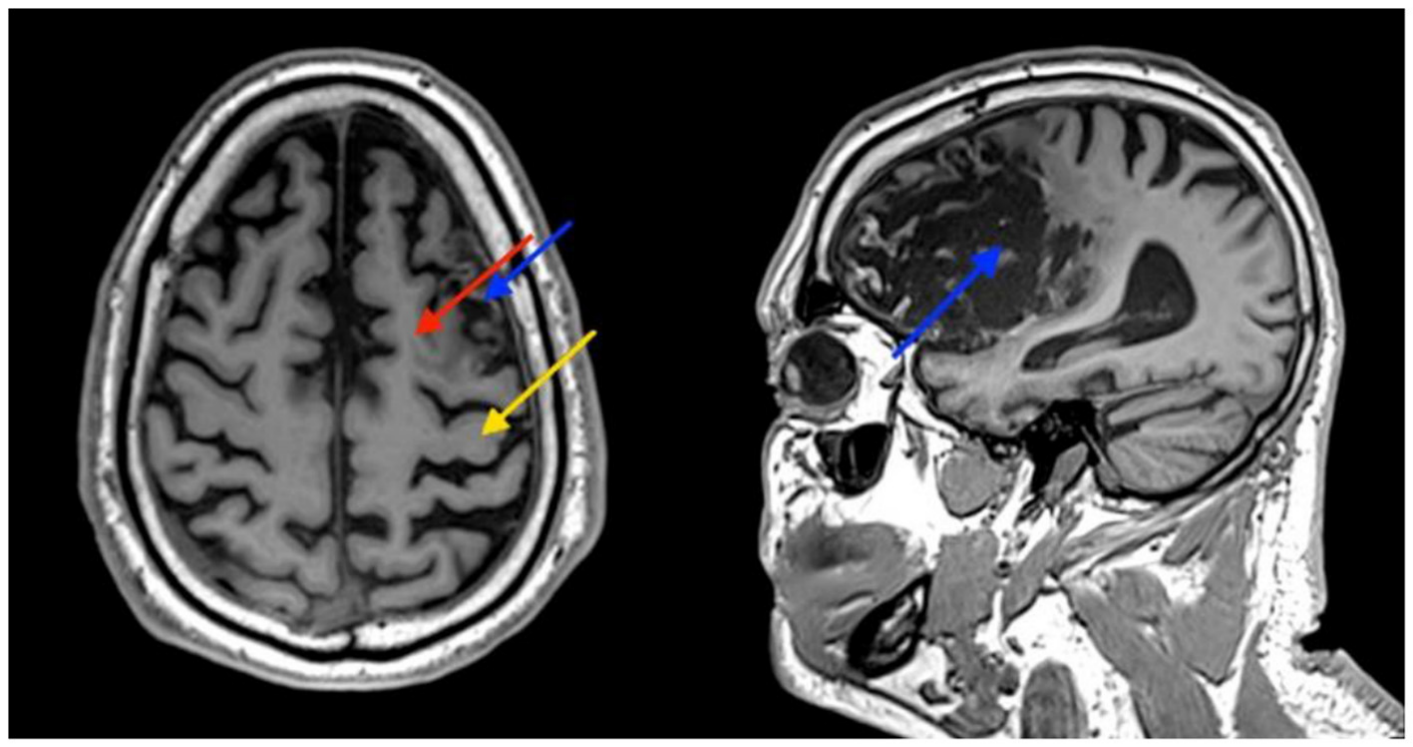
Patient's brain MRI. Images show atrophy and some damage to the supplementary motor cortex (SMA) (red arrow), and severe damage to the premotor cortex (PMA) (blue arrow). The primary motor cortex is relatively intact (yellow arrow).
